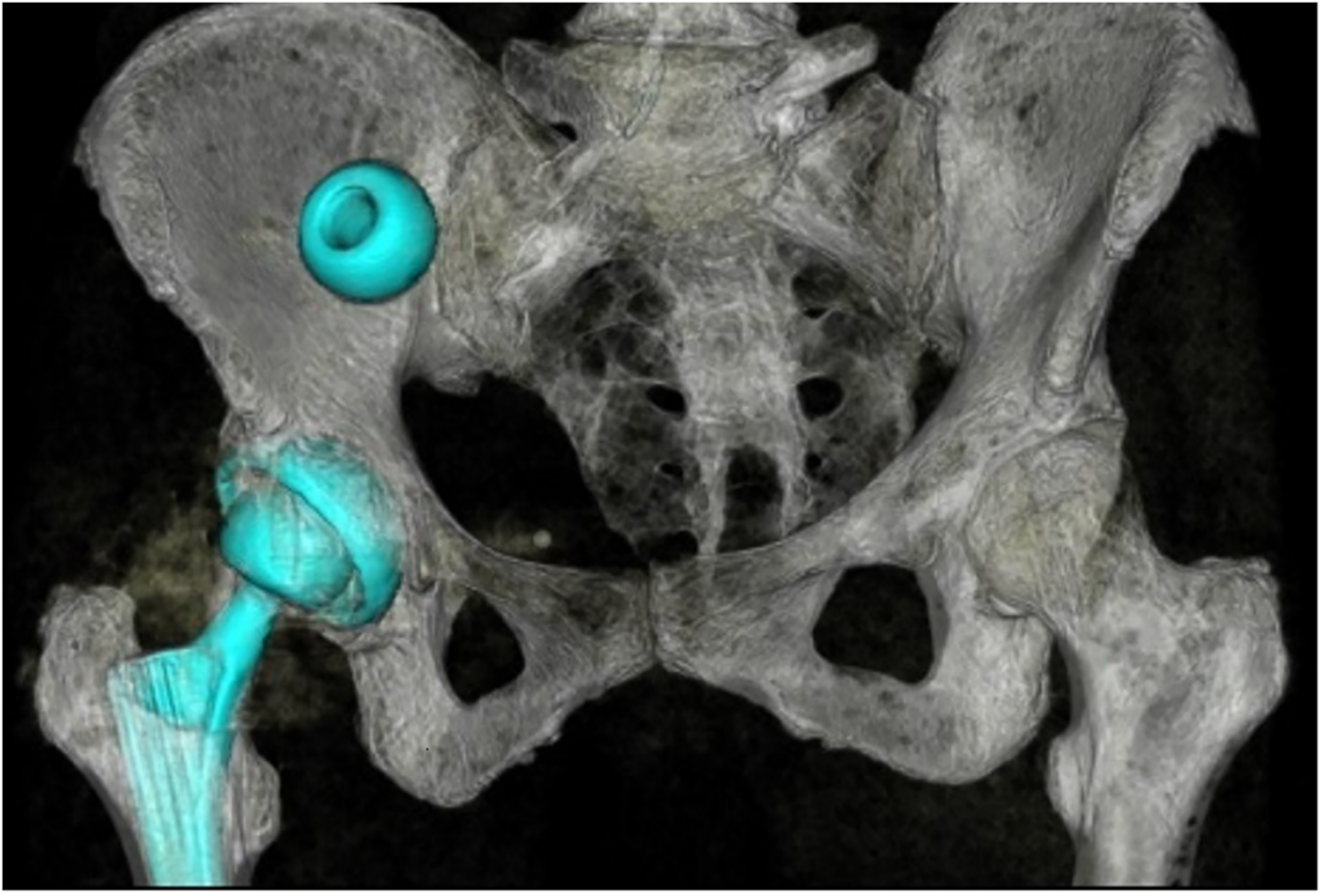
3D reconstruction of the pelvic CT scan.
